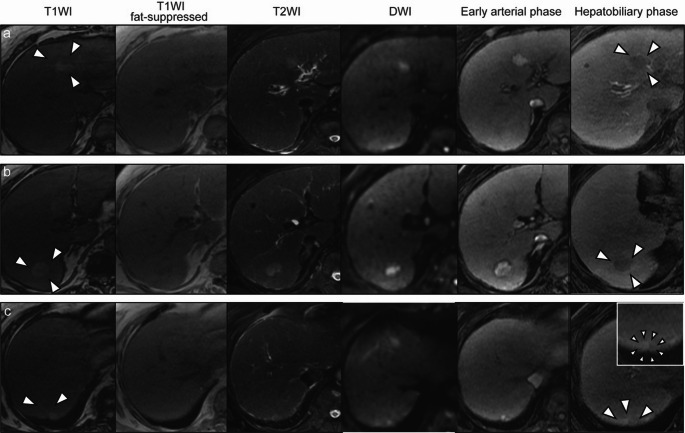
Magnetic resonance imaging (MRI) assessment of the S4, S6, and S7 nodules at the initial examination. Nodules in the a S4, b S6, and c S7 demonstrated the following characteristics: hyperintensity on T1-weighted imaging (T1WI), isointensity on fat-suppressed T1WI, hyperintensity on both T2-weighted imaging (T2WI) and diffusion-weighted imaging (DWI), early enhancement in the arterial phase. In the hepatobiliary phase, the S4 and S6 nodules demonstrated decreased uptake with low signal intensity, while the S7 nodule retained weakly enhanced signal intensity with less reduction in uptake. In panel (c), the top‑right inset provides the enlarged hepatobiliary phase view. White arrowheads indicate the nodules. Most of these imaging features were consistent with hepatocellular carcinoma (HCC)
